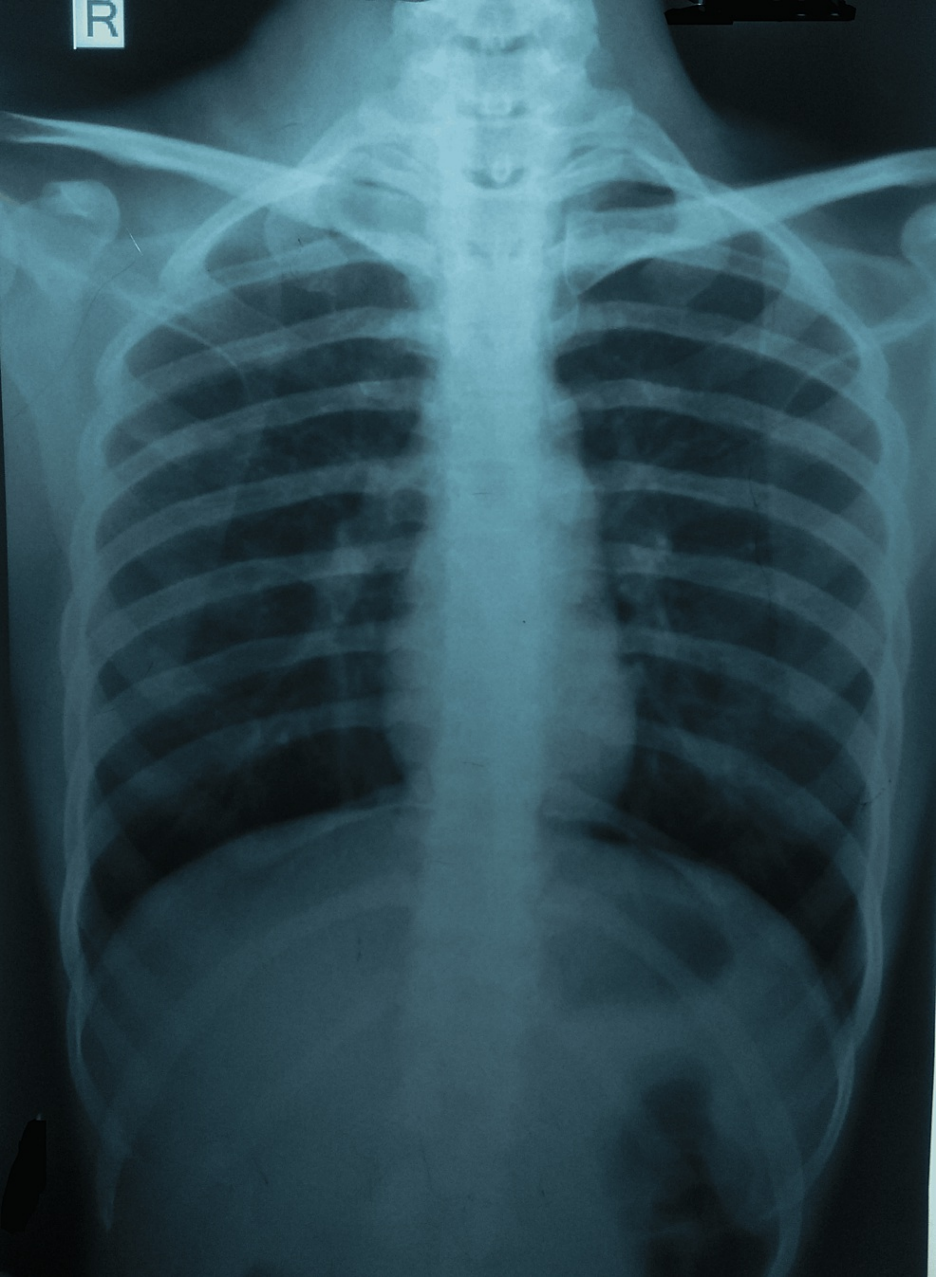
A normal chest radiograph
